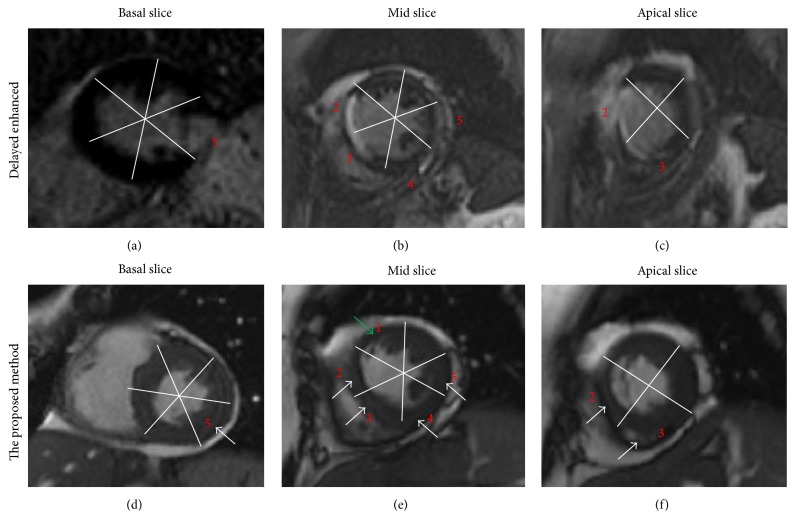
Comparison between (a–c) infarcted regions using delayed enhancement (DE) MRI and (d–f) regions with motion abnormality detected using the proposed method.
